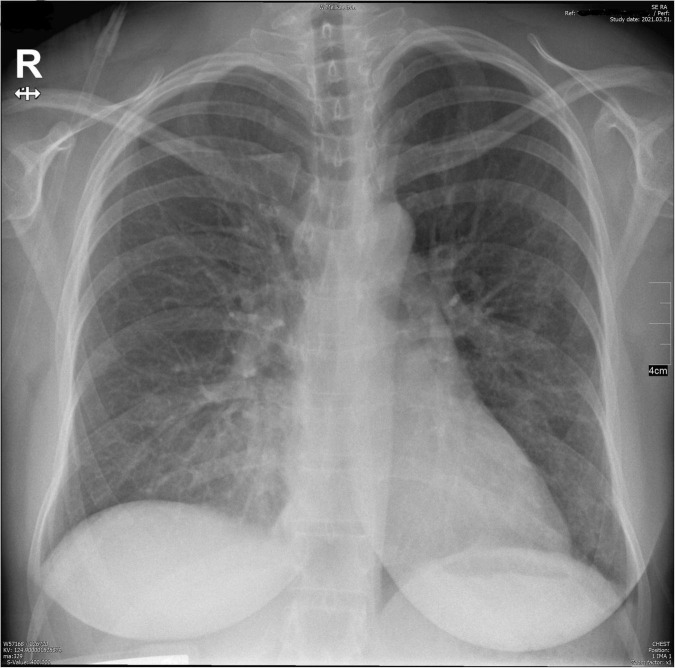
Pulmonary X-ray on the 6th day.
